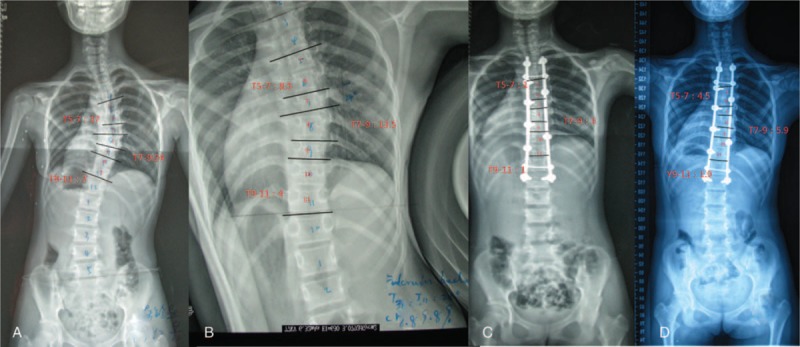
Cobb angle of the proximal (T5–T7), apical (T7–T9), and distal (T9–T11) segments of the main thoracic curve (T5–T12) is measured. A, B, C and D indicate the posteroanterior X-ray film before surgery, fulcrum-bending film and posteroanterior film 5 days after surgery, and posteroanterior film at the end of the 3-year follow-up period, respectively.
